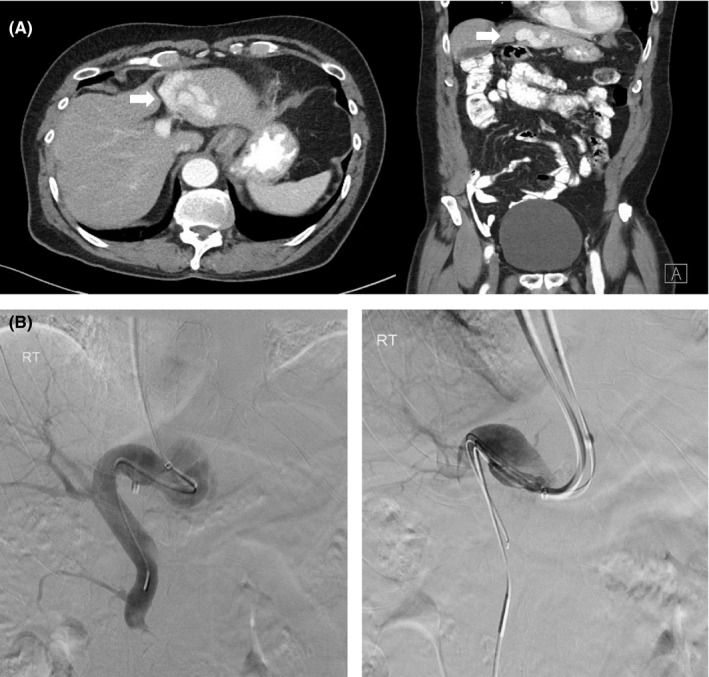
A 72‐year‐old man with congenital intrahepatic portosystemic venous shunt. A, Axial and coronal images from the pre‐embolization contrast‐enhanced CT of the abdomen and pelvis which demonstrates the abnormal enlarged intrahepatic veins corresponding to left portal vein to left hepatic vein shunt (white arrow). B, Portal venography with fluoroscopic DSA obtained using transjugular access through the left hepatic vein into the main portal vein across the spontaneous shunt
